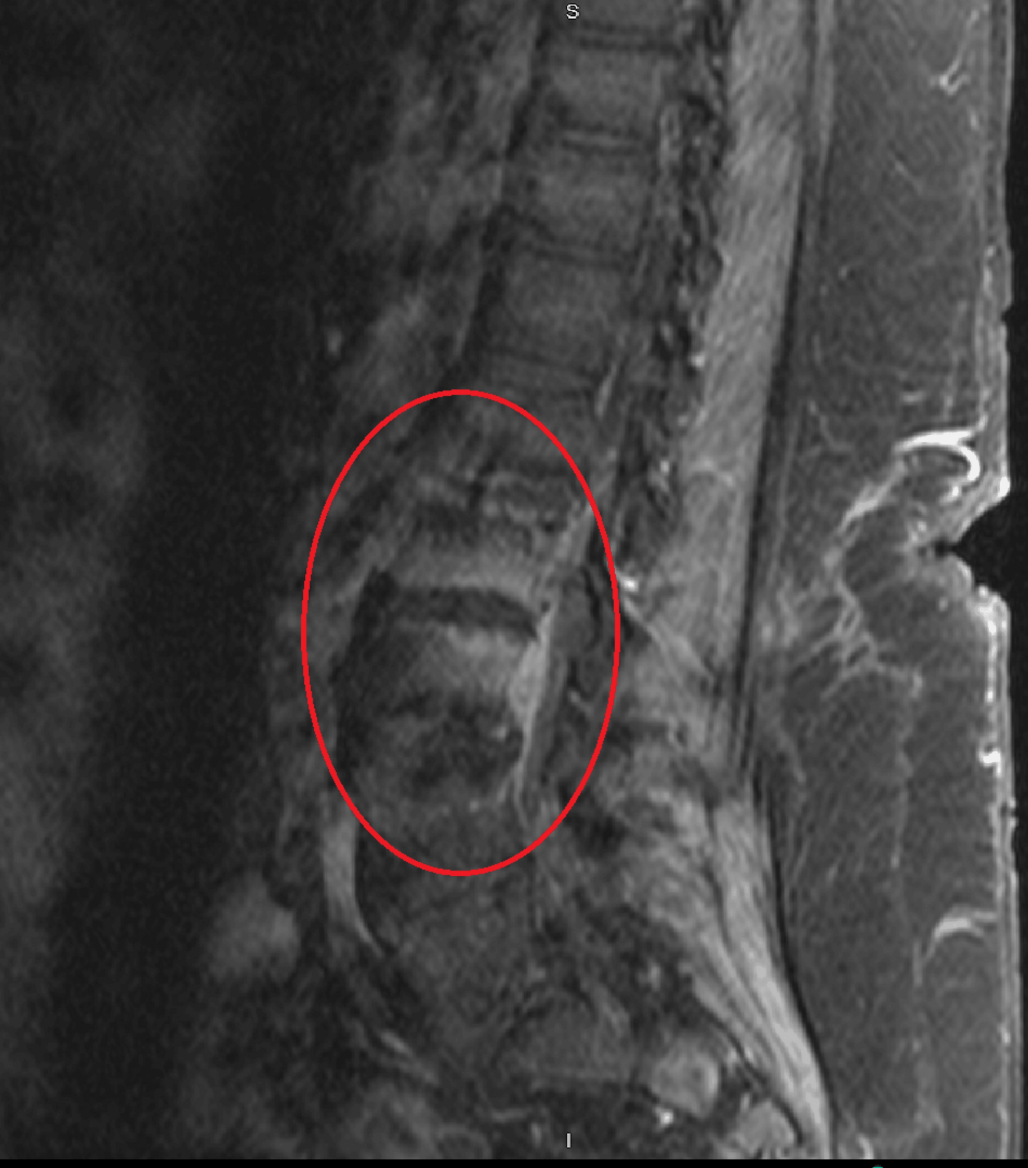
MRI spine lumbar w + wo contrast MRI: magnetic resonance imaging The red circle indicates spondylodiscitis and concerns of osteomyelitis, particularly severe degenerative disc disease at L1-L2 without posterior disc height and mild to moderate degenerative disc disease at L2-L3 with extensive vertebral body end plate edema
